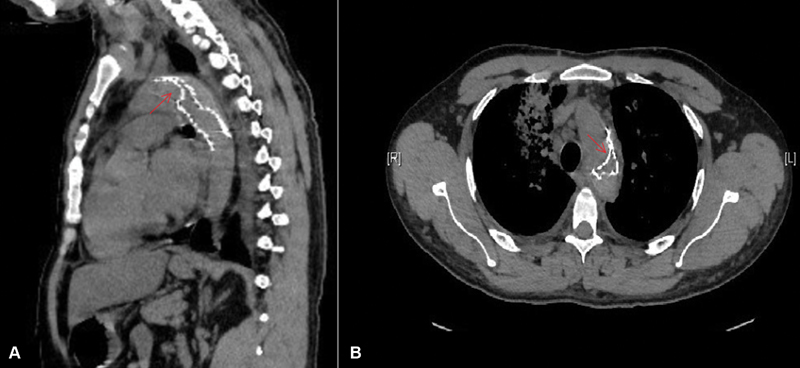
Computed tomography for chest showed inner (medial) wall collapse of the stent graft inserted in traumatic aortic transection as showed in arrow in sagittal view (
